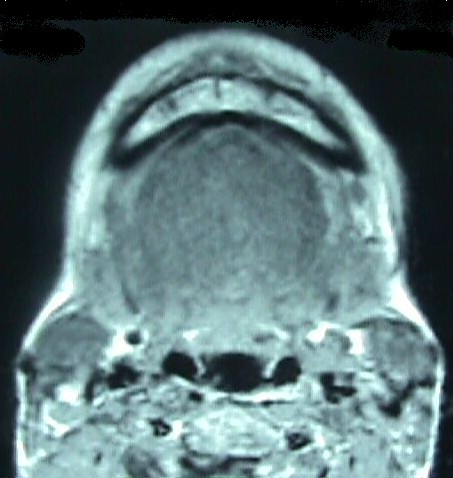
A MRI scan showing an 40 × 35 mm well-circumscribed non-enhancing cystic mass extending from the sublingual area to the thyroid notch level.
